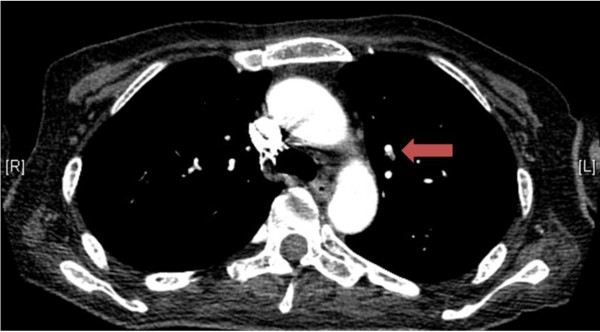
Contrast-enhanced CT scans of the lung. A small pulmonary embolism in the left upper lobe was identified.
